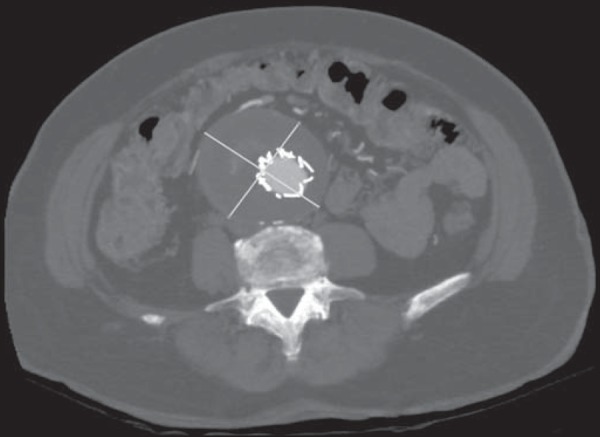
Axial section CT angiography showing the measuring of the aneurysm sac.
